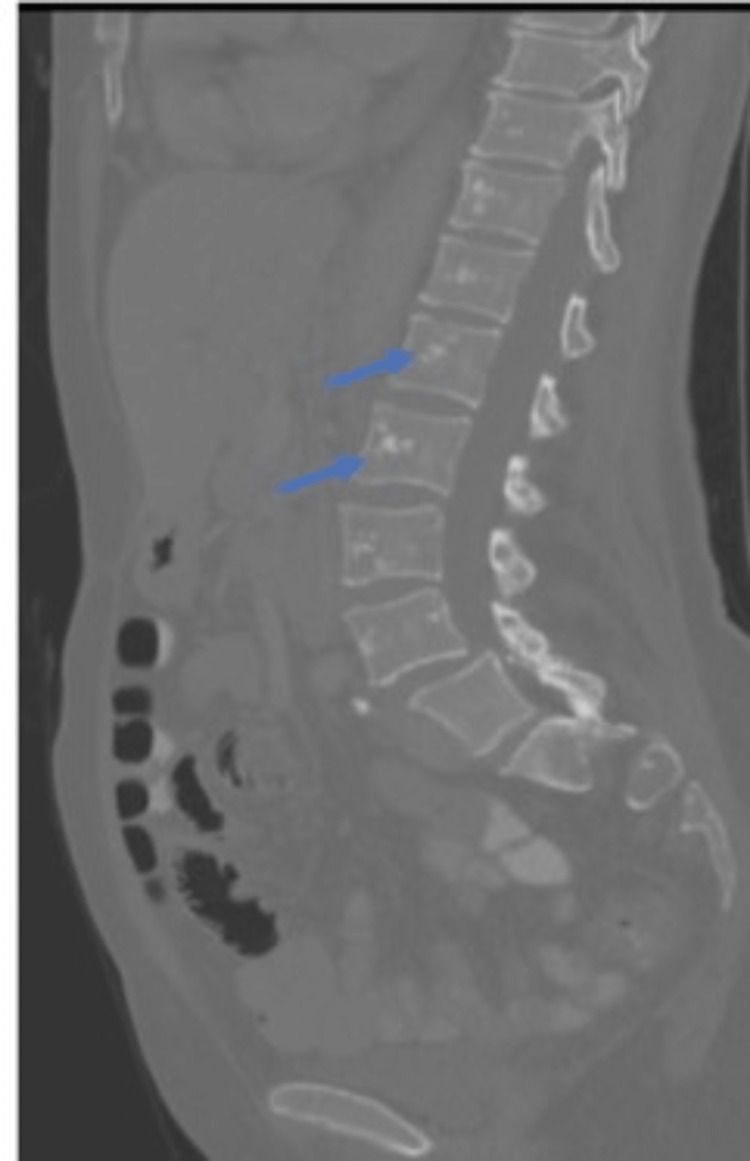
Computed tomography of the abdomen and pelvis showing multiple sclerotic lesions (blue arrows) in the thoracic and lumbar spine.
